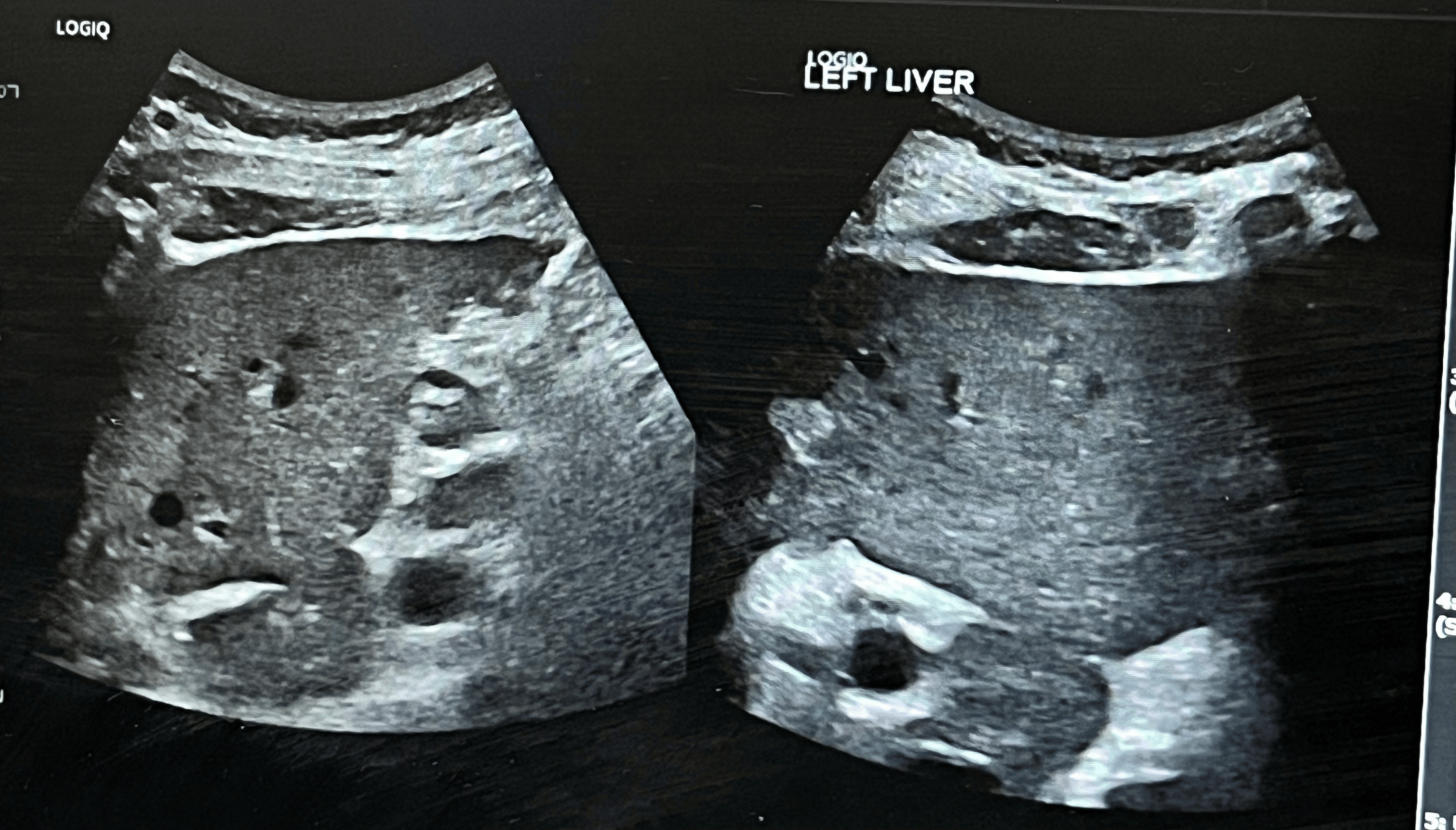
Ultrasound image showing no signs of cirrhosis or bile duct obstruction.
